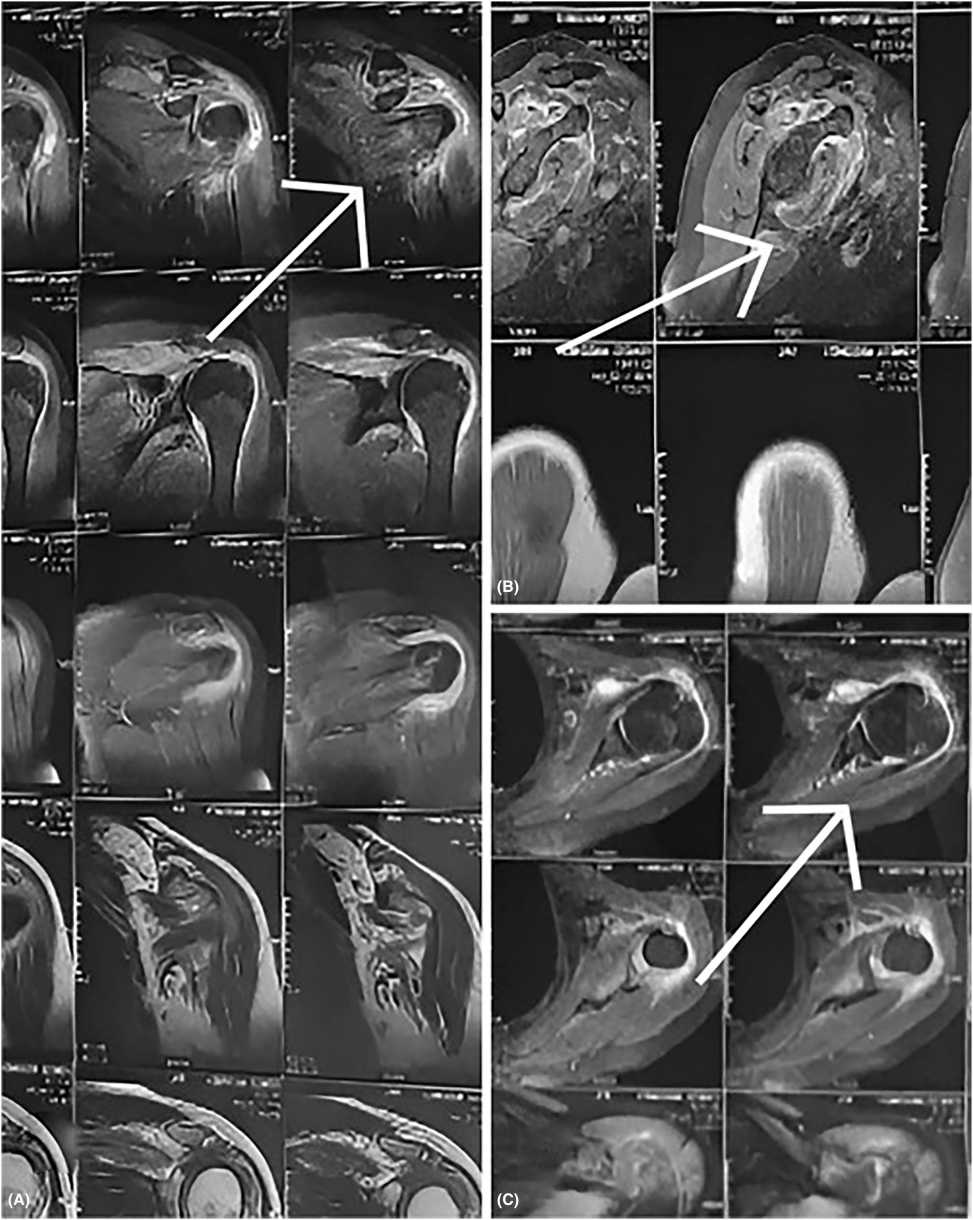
MRI related to table number two, which shows a high‐grade tear with muscle edema and strain in the supraspinatus tendon (A), an anterosuperior labral tear in the glenoid labrum (B), and a partial tear of the IGHL glenohumeral ligaments (C).
